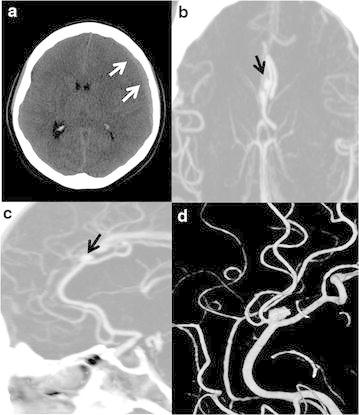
a Non-contrast brain CT shows acute subdural hematoma in the left fronto-tempo-parietal area (*white arrow*). The *midline* is shifted about 6 mm to the right. b, c Brain CTA reveals an A3–A4 junction aneurysm (*black arrow*). d 3D-DSA reveals an aneurysm at the A3–A4 junction
